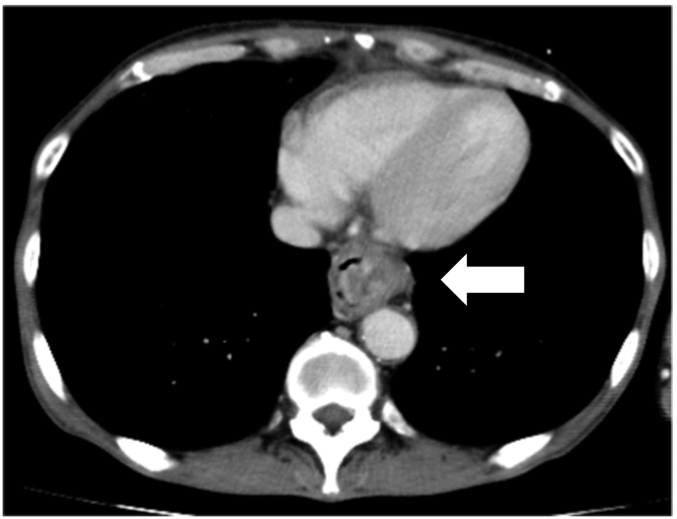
Enhanced computed tomography image. Tumor visualized in the lumen of the lower-third thoracic esophagus (arrow).
